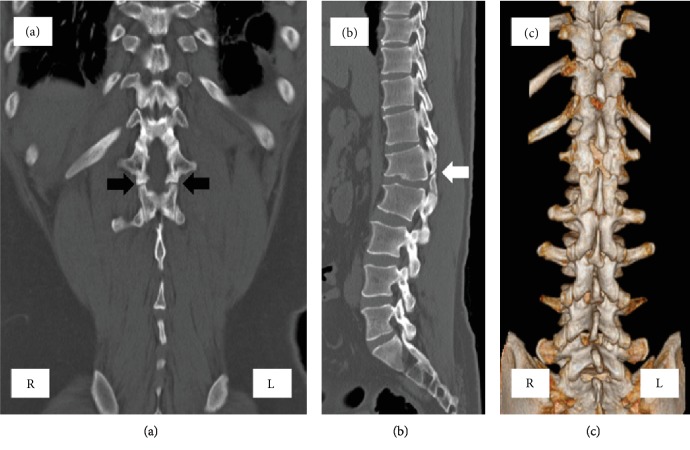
Computed tomographic (CT) scan. Coronal view (a) revealed bilateral spondylolysis (black arrow) and a bony defect of the lamina at L1. A sagittal view (b) revealed spondylolysis (white arrow) at L1. Three-dimensional reconstruction of the computed tomography scan (c) showing the coexistence of spina bifida and spondylolysis at L1. R indicates right side; L, left side.
